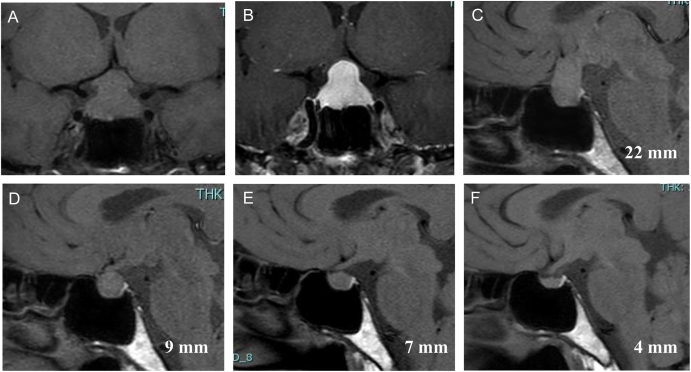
Temporal evolution of MRI findings on the pituitary gland. T1-weighted image, frontal (A) and sagittal (C) views taken 3 days postpartum. Gadolinium-enhanced image, frontal view (B), 3 days postpartum. T1-weighted image, sagittal view captured at 3 months (D), 6 months (E), and 17 months (F) after the start of treatment. The height of the pituitary gland was indicated in MRI images in (C), (D), (E), and (F).
